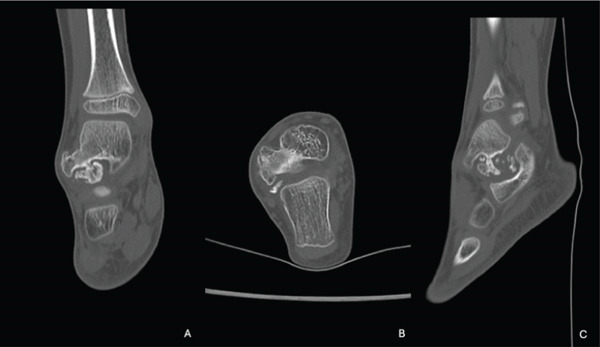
Coronal (A), axial (B), and sagittal (C) CT images demonstrating cortical and medullary continuity between the lesion and the lateral talar body, with separation from the articular surface, supporting metaphyseal origin.
